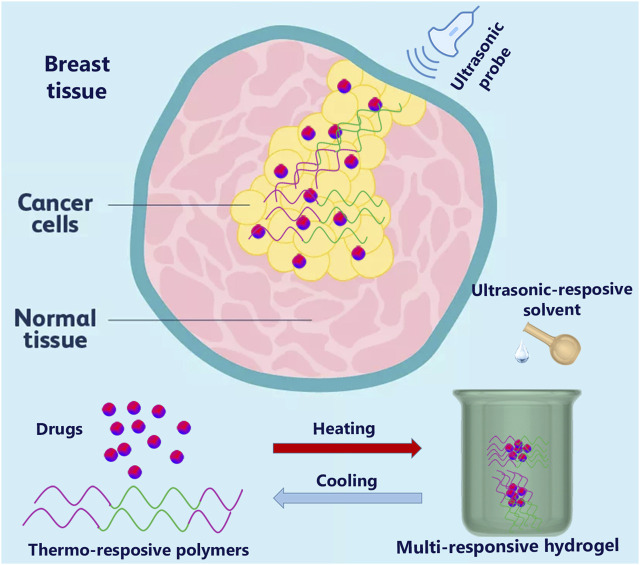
The multi-responsive hydrogel based on temperature and ultrasound triggers for breast cancer.
